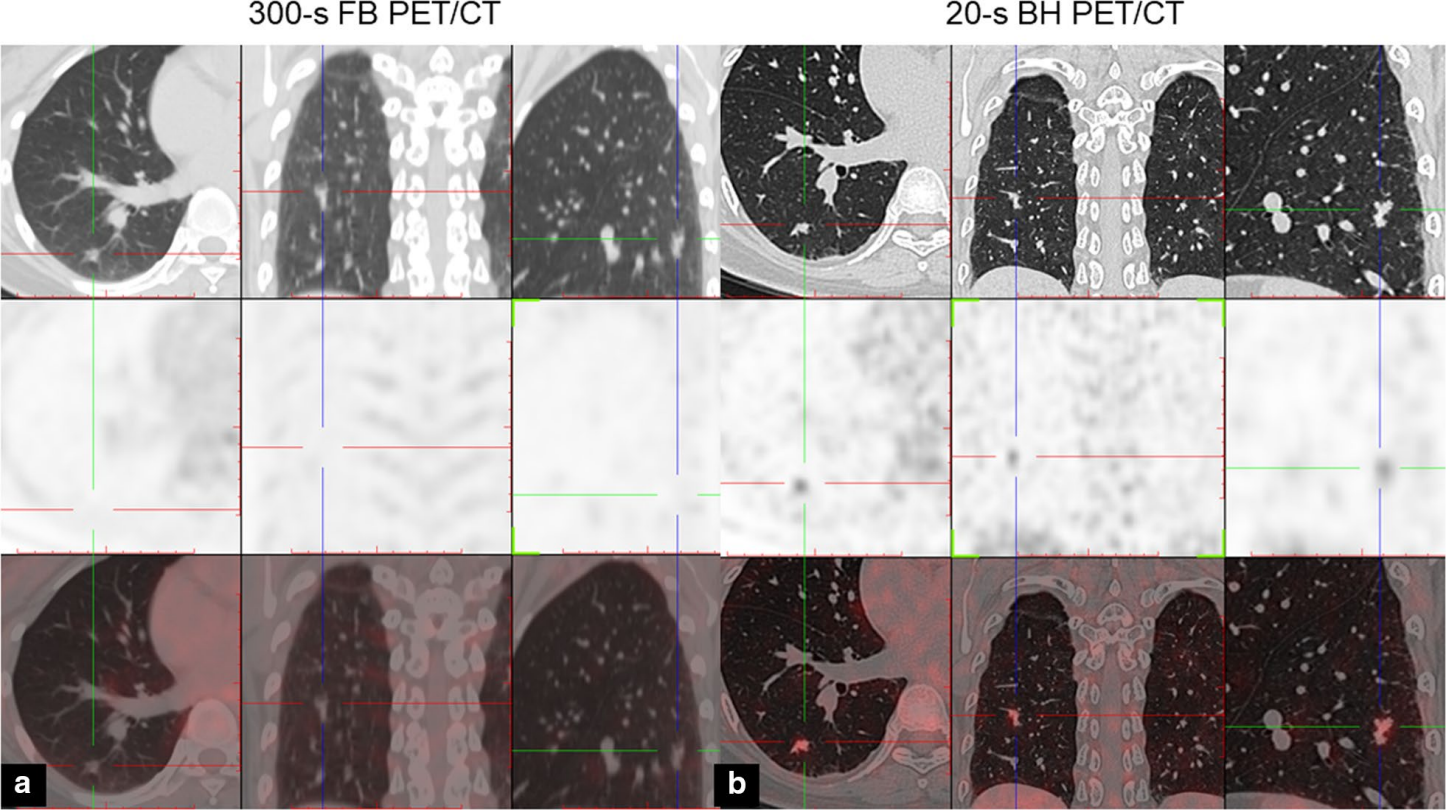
44-year-old woman with lung adenocarcinoma. Axial, coronal, and sagittal chest CT (top row), PET (middle row) and PET/CT (bottom row) images of solitary solid lung nodule in right lower lobe. a FB PET/CT; b BH PET/CT. Maximum axial diameter of the lesion was 9.2 mm. Lesion was not clearly visualized on FB PET but was conspicuous on BH PET
